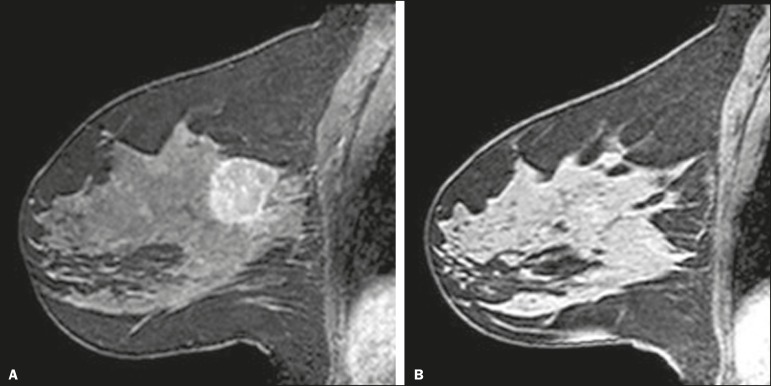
A: Pre-NAC contrast-enhanced sagittal DCE-MRI sequence showing a nodule with a mildly irregular form and ill-defined margins, with heterogeneous internal enhancement (invasive ductal carcinoma proven by core biopsy). B: Post-NAC contrast-enhanced sagittal DCE-MRI sequence not showing the previous nodule in which a pCR, according to the Response Evaluation Criteria in Solid Tumors criteria, was achieved.
